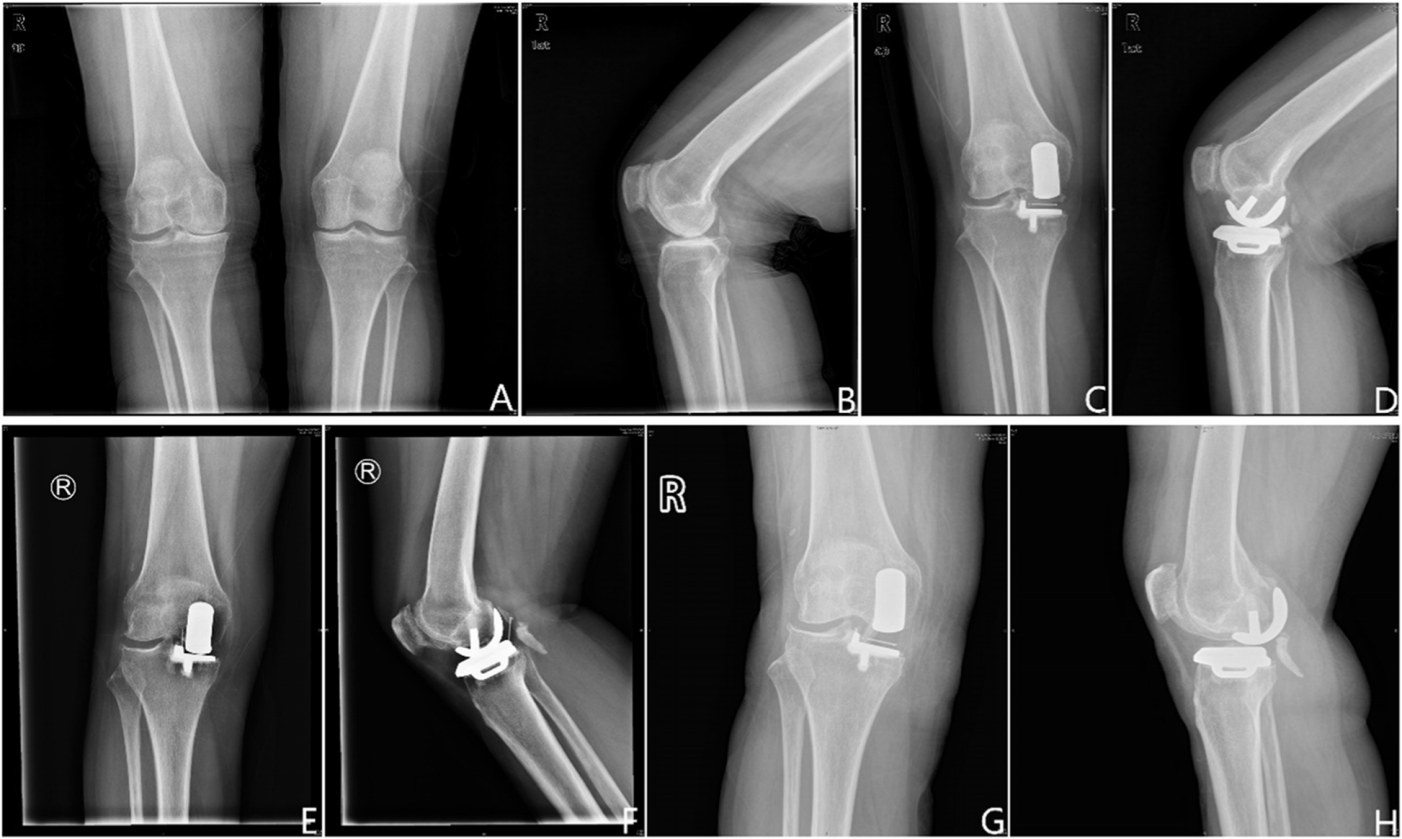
A, B Preoperative radiographs of the patient. C, D Postoperative radiographs of the patient after primary UKA. E, F Posterior bearing dislocation after primary UKA. G, H Thicker bearing exchange after bearing dislocation
